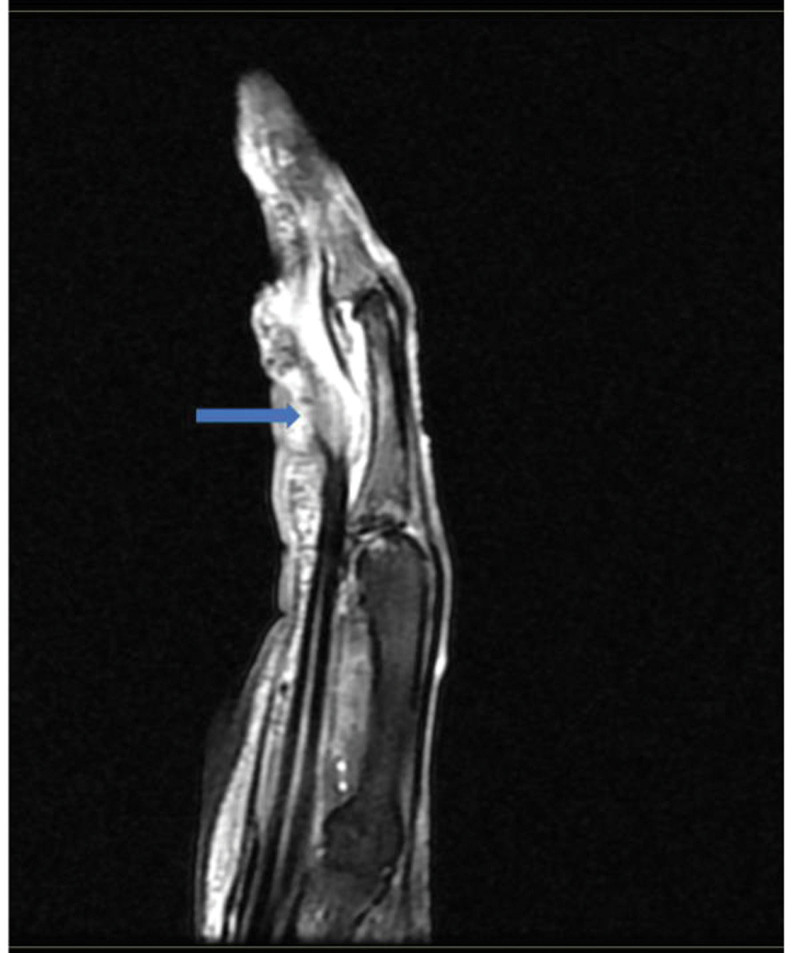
Sagittal magnetic resonance imaging (MRI) of the right middle finger. The blue arrow indicates the rupture of the flexor tendon.
